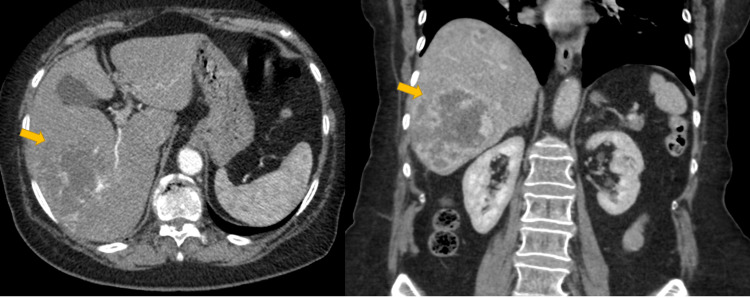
HA mimicking cavernous haemangioma Axial and coronal imaging of CECT showing the lesion with appearances in keeping with cavernous haemangioma (yellow arrow). Interpretation of the lesion as being a cavernous haemangioma was misleading, as the lesion was representing an HA. HA: hepatic angiosarcoma; CECT: contrast-enhanced computed tomography
